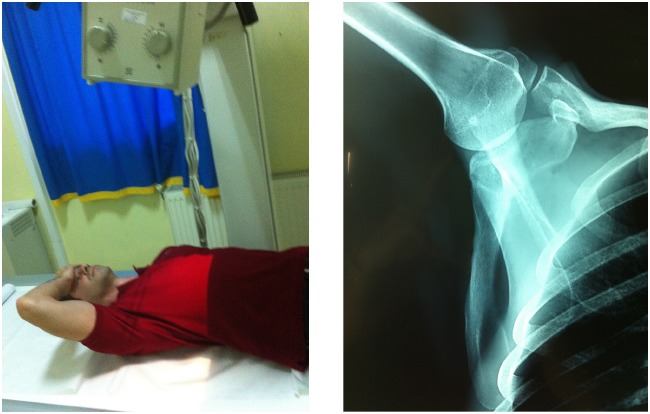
a. Stryker notch view: the patient is supine, placing the palm of his affected hand on top of his head and the beam is tilted 15° cephalad. b. A stryker notch radiograph of the right shoulder.
